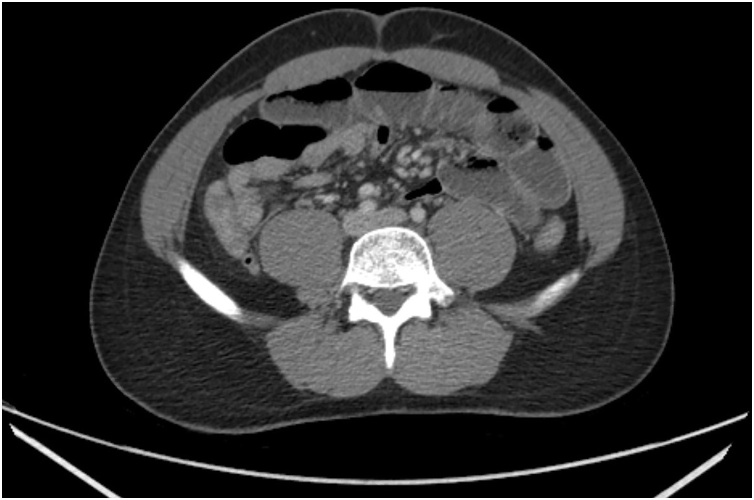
Axial view of CT abdomen and pelvis on admission.
